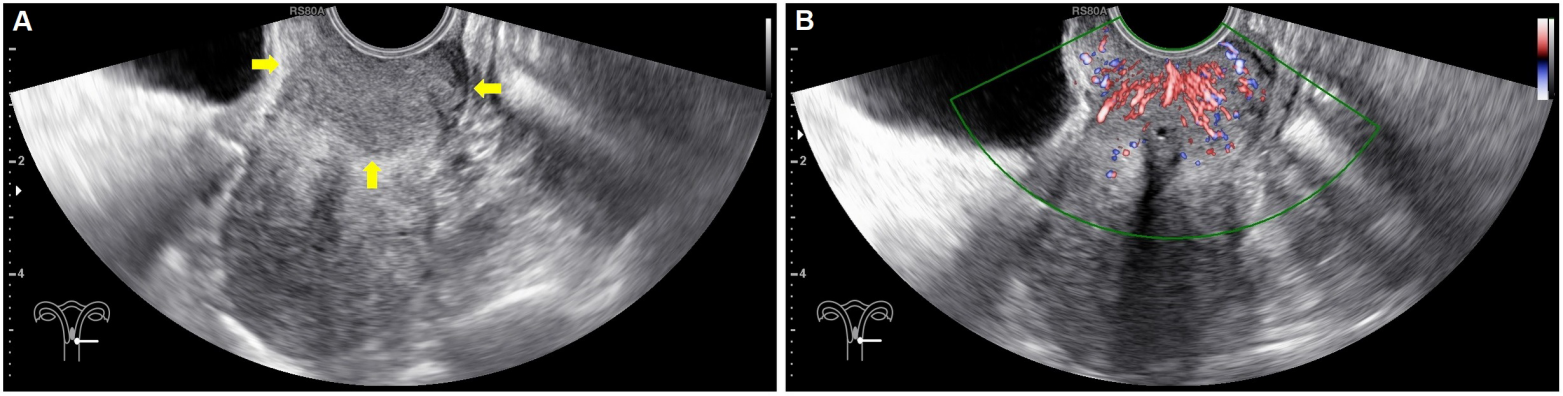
Baseline ultrasonography and color Doppler flow imaging (CDFI) in a 41-year-old with confirmed stage IIB cervical cancer. (A) Sagittal gray-scale images of the uterus reveal a hypoechoic, well-defined a lesion (arrows) in the cervix, with tortuosity of the cervical canal and unclear visualization. (B) Colour Doppler flow imaging showed spotted intratumoral blood flow signals.
